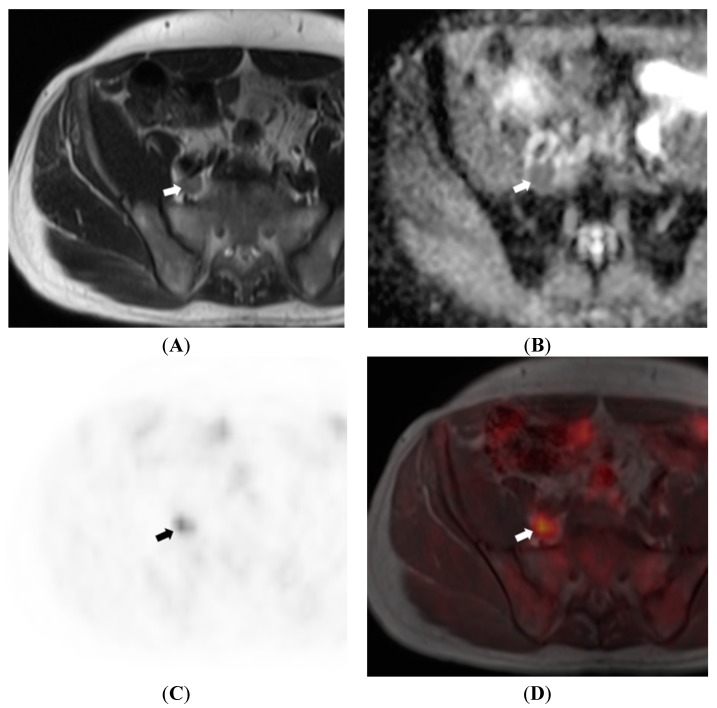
64 year old male patient with prostate cancer. T2WI (A) show a large right common iliac lymph node (arrow) which exhibits restricted diffusion on ADC map (B); ^18^F-choline PET (C) and fusion PET/MRI (D) confirm the lymph node to be metabolically active, further increasing confidence regarding it being metastatic.
